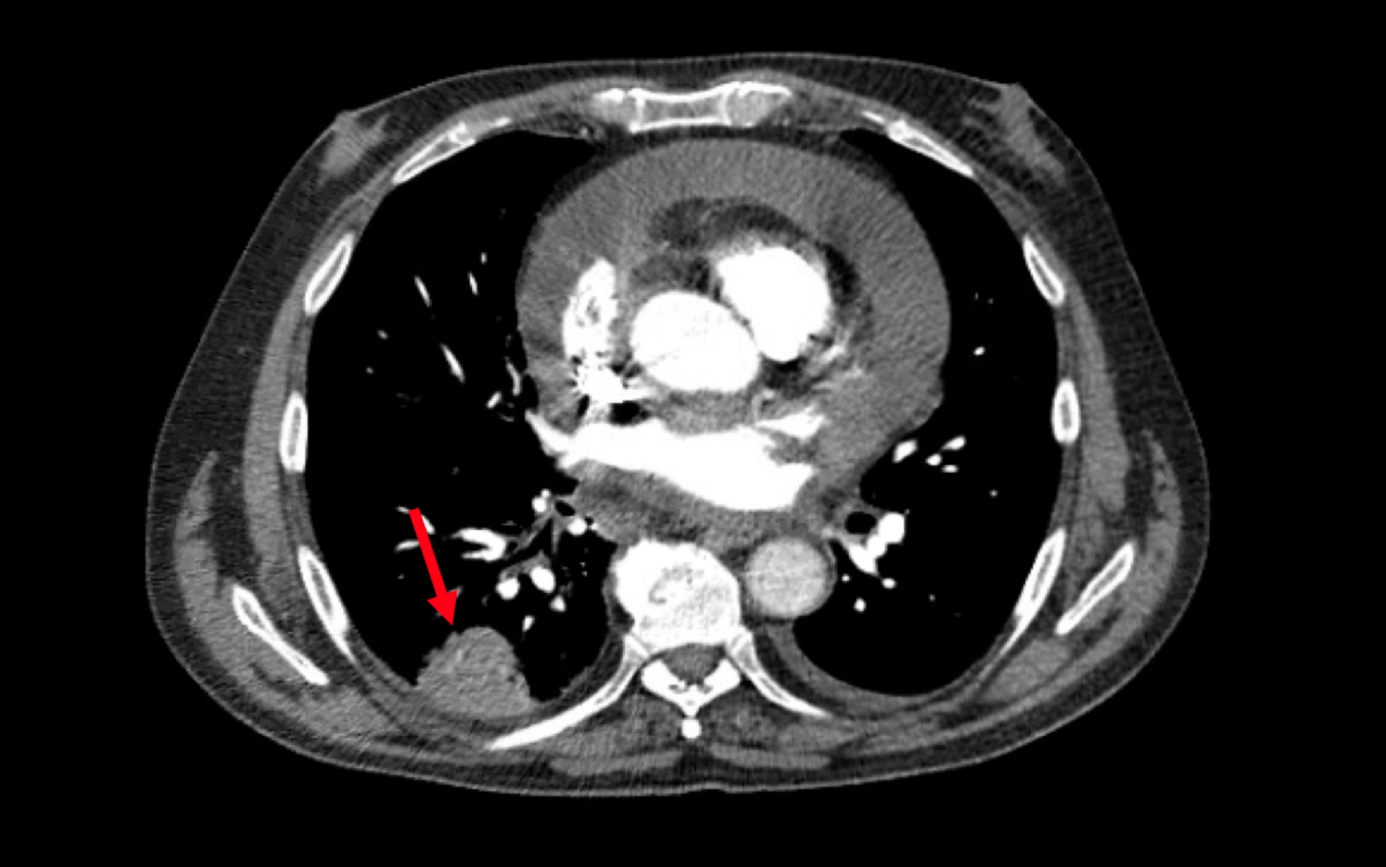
Computed tomography of the chest with the arrow pointing towards a right basilar pulmonary nodule
